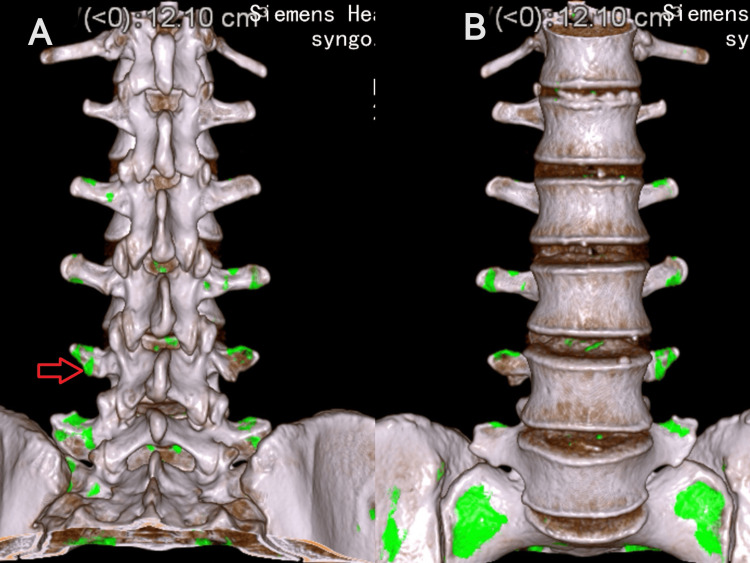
Dual-energy CT for post-treatment evaluation (A) 3D posterior aspect of the lumbar spine; (B) 3D anterior aspect of the lumbar spine. The green area indicated by the red arrow shows the urate deposits, with a volume of approximately 12.10 cm³.
